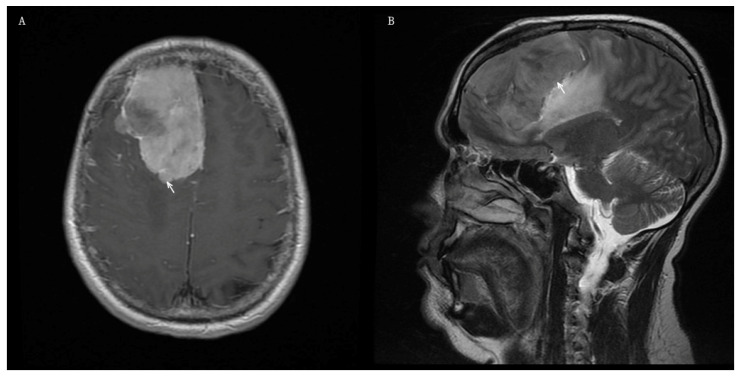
Preoperative MRI of Patient 3 demonstrating a RIM located in the right frontal lobe. White arrows indicate the tumors. (A) Axial T1-weighted image after gadolinium administration showing intense contrast enhancement of the lesion. (B) Sagittal T2-weighted image demonstrating peritumoral brain edema.
